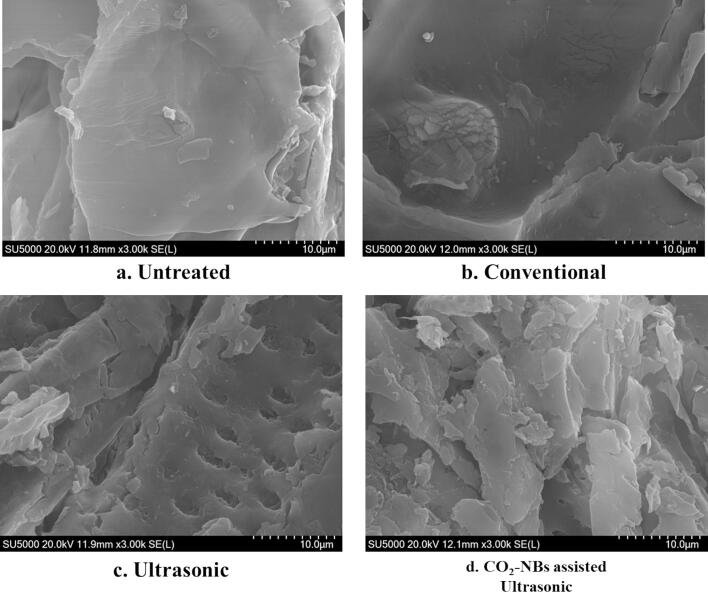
SEM images of peanut shell (PS) after extraction treatments (a) Untreated, (b) Conventional, (c) Ultrasonic, (d) CO_2_-NBs assisted ultrasonic.
